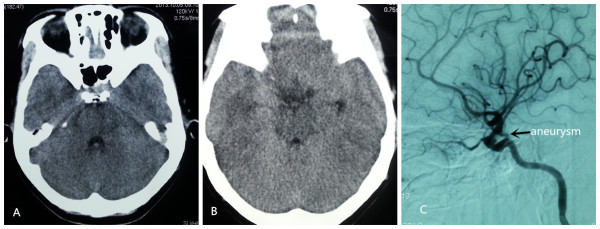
Pre-operative computed tomography of the brain with cerebral angiogram. (A, B) A pre-operative brain computed tomography scan demonstrated a small amount of increased signal in the basal cisterns; no evidence of intrasellar and suprasellar lesions was seen. (C) Angiogram shows a left posterior communicating artery aneurysm (arrow).
